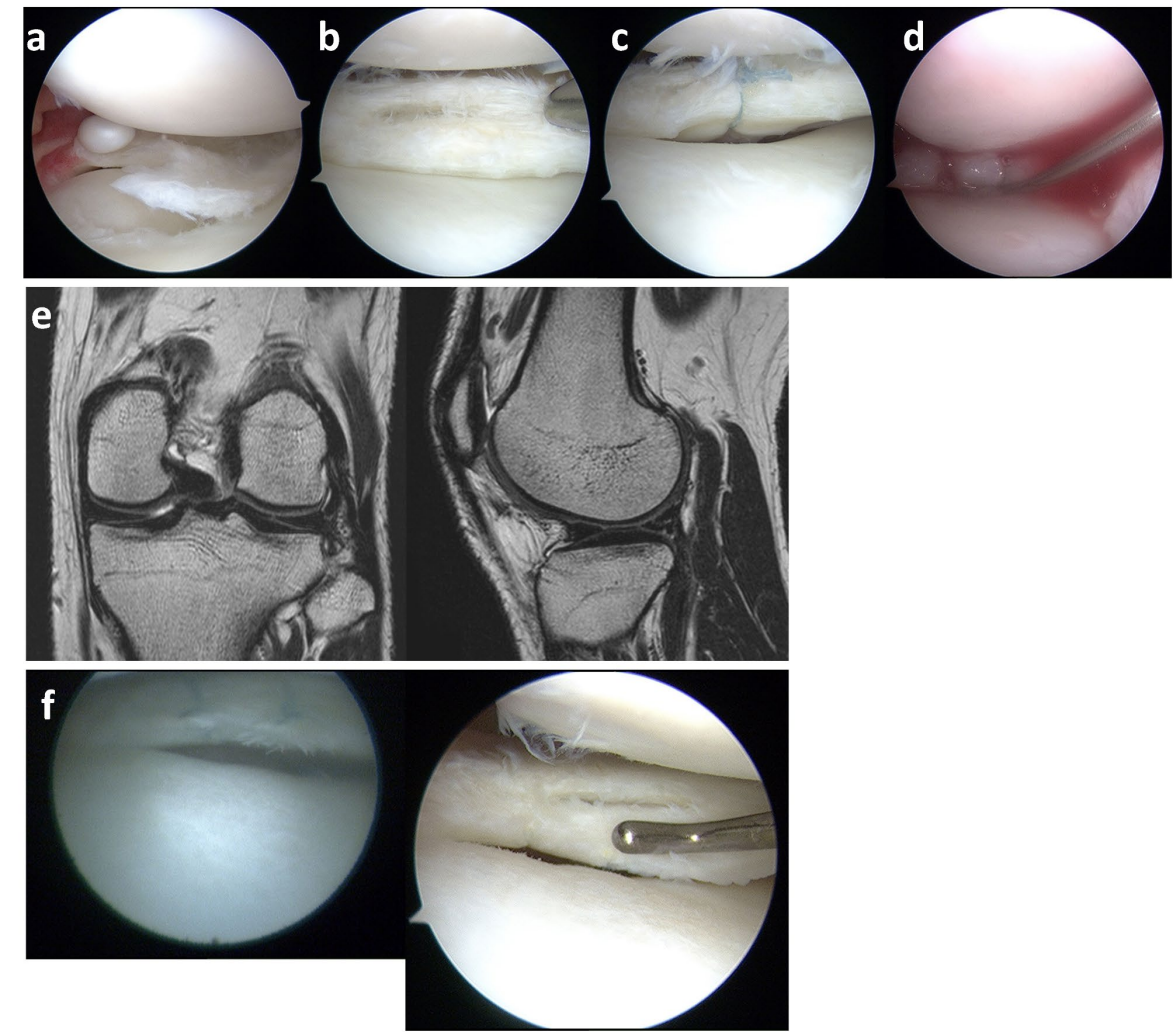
Treatment course of patient #4. (a) The left lateral meniscus shows a discoid meniscus with a flap tear in the posterior segment. (b) Following partial meniscectomy, a horizontal tear with degenerative changes is seen in the posterior segment. (c) Vertical sutures were placed arthroscopically using an all-inside device, followed by (d) P47K-WAS-MR gel application with a needle. (e) Postoperative T2-weighted MRI shows grade 2 healing in coronal and sagittal views. (f) Second-look arthroscopy shows an incompletely healed meniscus with views from the Needle Arthroscope^®^ (left) and conventional arthroscopy (right); while sutures are visible, the Needle Arthroscope^®^’s lower resolution limits probing of the repair site.
